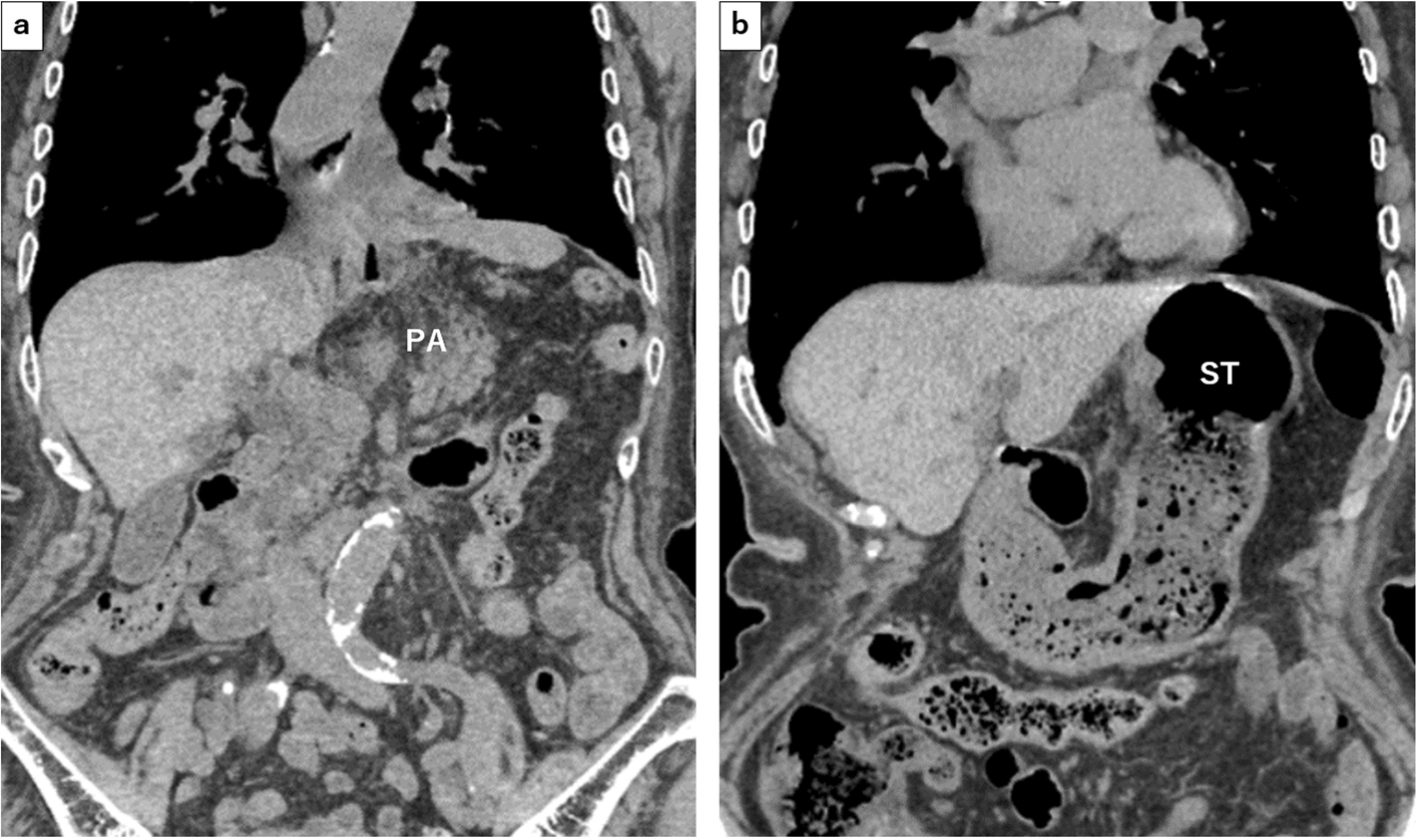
a, b CT on day 30 after the repair of the HH showed that the stomach and pancreas were located in the abdomen. PA: pancreas, ST: stomach
